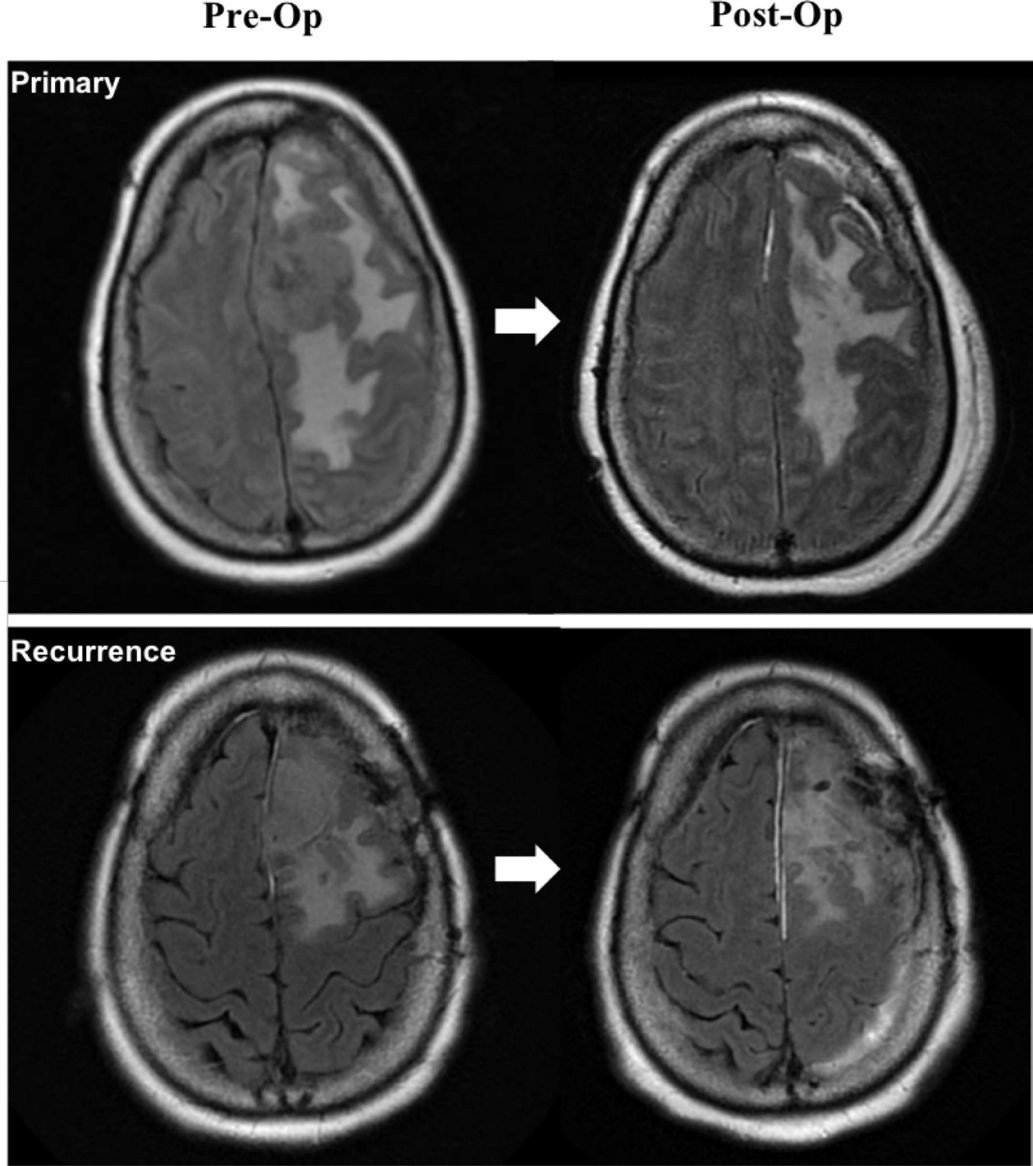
T2 FLAIR magnetic resonance (MR) imaging of a representative patient with an *NF2* mutation positive WHO grade II meningioma (frameshift deletion at locus chr22:30074229) showing large tumor volume with vasogenic edema for the primary tumor (*top*) and a recurrence (*bottom*). Left images depict preoperative MR imaging and right images are postoperative MR imaging. Recurrent meningioma was found to have an *ARID1A* nonsense mutation at locus chr1:27101099 in addition to the *NF2* frameshift deletion.
